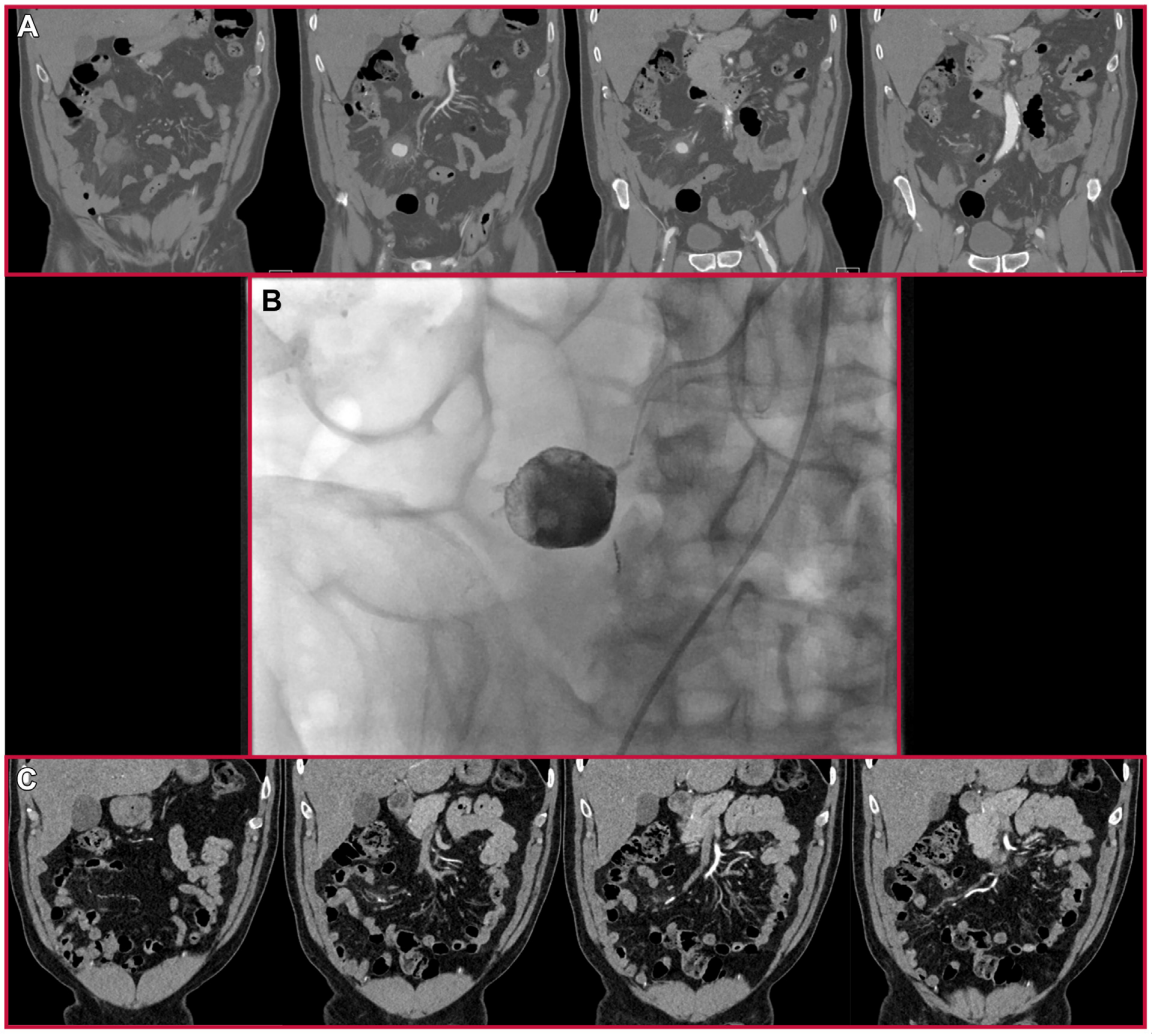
Ileocolic artery pseudoaneurysm that presented on readmission. (A) Diagnostic computed tomography (CT) angiogram revealing contained pseudoaneurysm. (B) Angiogram and coiling in the interventional radiology suite. (C) Review of index diagnostic CT angiogram revealing mesenteric inflammation.
